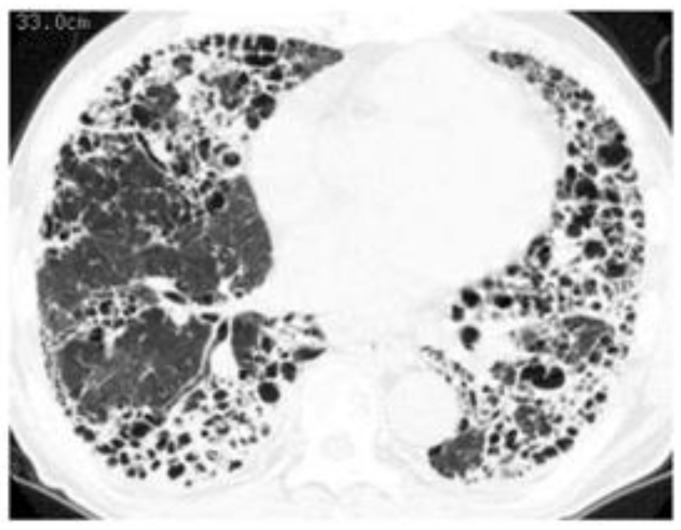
A case of RA-ILD in which the complication of nontuberculous mycobacterial pulmonary disease (*Mycobacterium kansasii* infection) developed. Chest computed tomography showed consolidation along with honeycombing.
